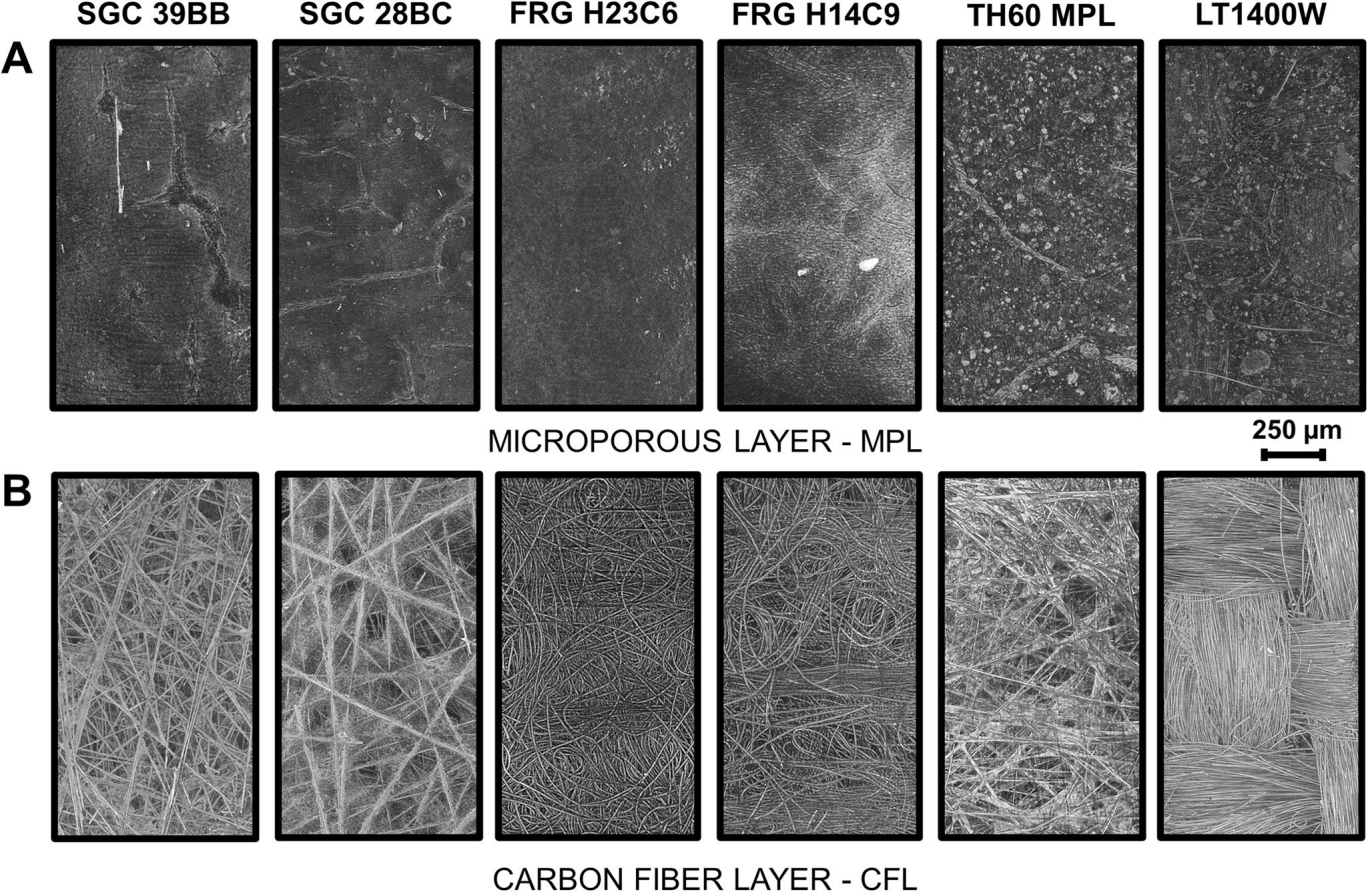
Structure of different commercially available carbon gas diffusion layers. X-ray computed tomography of selected gas diffusion layers: (A) shows the side covered by the microporous layer, while (B) shows the other side.
